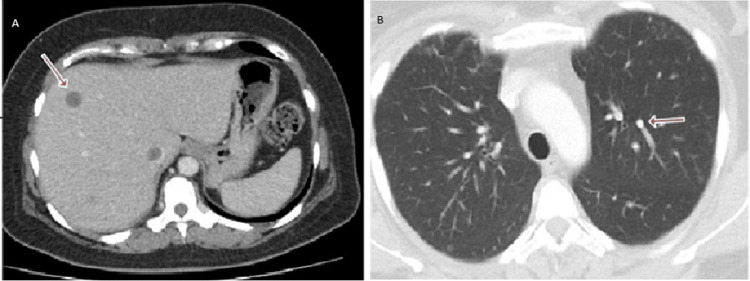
CT scan showing (A) Interval reduction in size of hepatic metastasis; (B) stable pulmonary metastasis.
